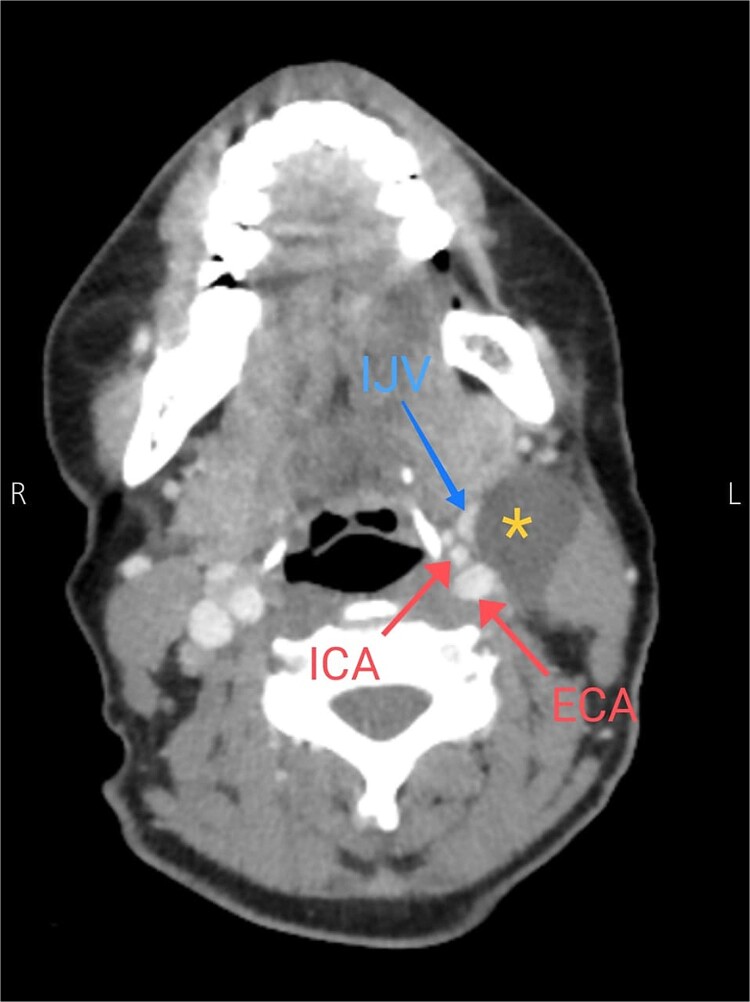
Axial CT neck scan showing the branchial cleft cyst compressing the IJV and adjacent to the external and internal jugular arteries. Asterisk: branchial cleft cyst, arrows: external and internal carotid arteries, blue arrow: IJV*.*
